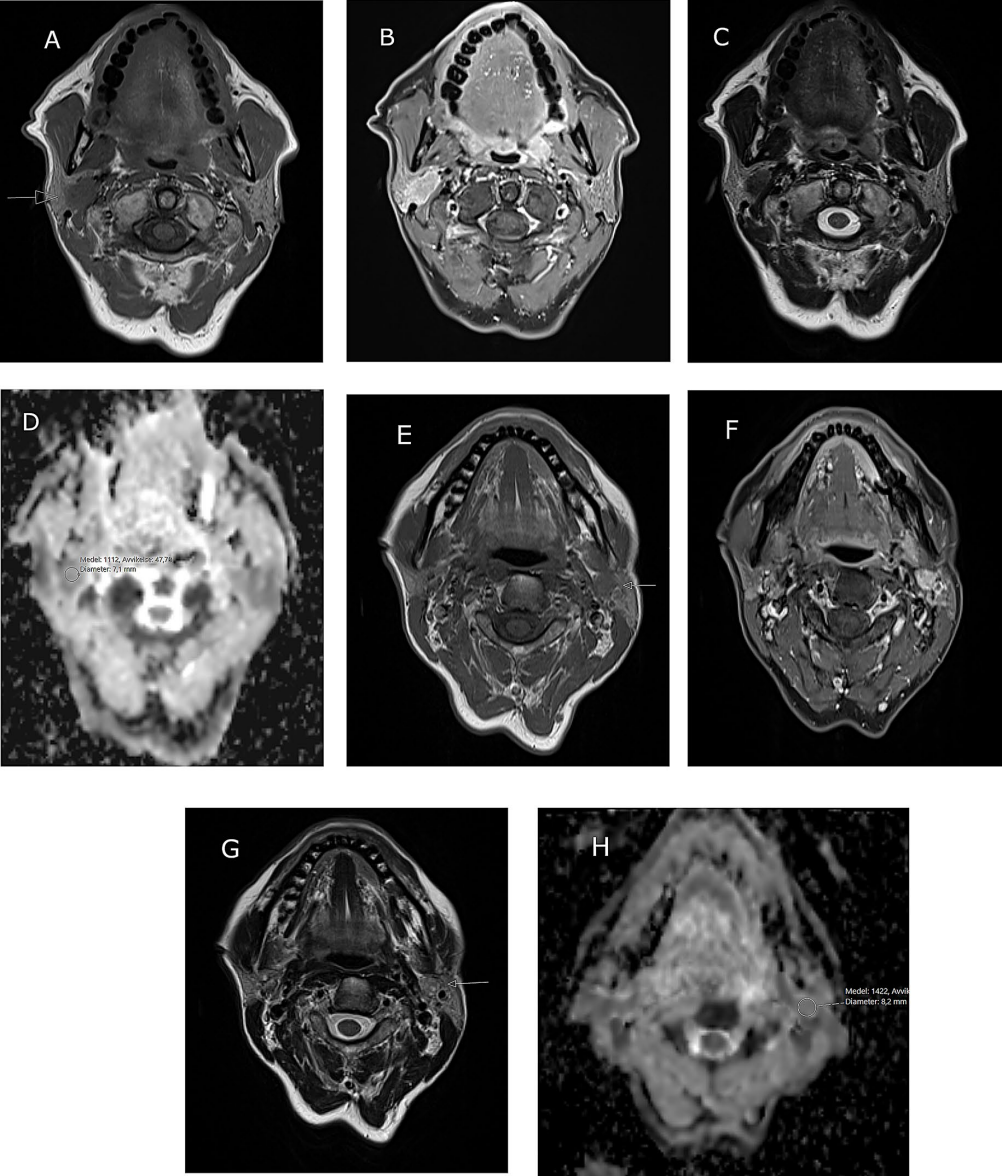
Patient 1, MR image of bilateral salivary gland tumors in a 56-year old female. A-D show a right sided intraparotid lesion with irregular borders. A) Low signal on T1W (arrow), B) rich contrast enhancement on fat-suppressed TIW, C) low signal on T2W and D) restricted diffusion on the ADC-map, ADC = 1,11 × 10^− 3^ mm^2^/s. E-H Left sided intraparotid lesion in the deep lobe. E) Low signal on T1W (arrow), F) rich contrast enhancement on fat-suppressed TIW, G) mixed signal on T2W (arrow) and H) moderate diffusion restriction on the ADC-map, ADC = 1,42 × 10^− 3^ mm^2^/s
